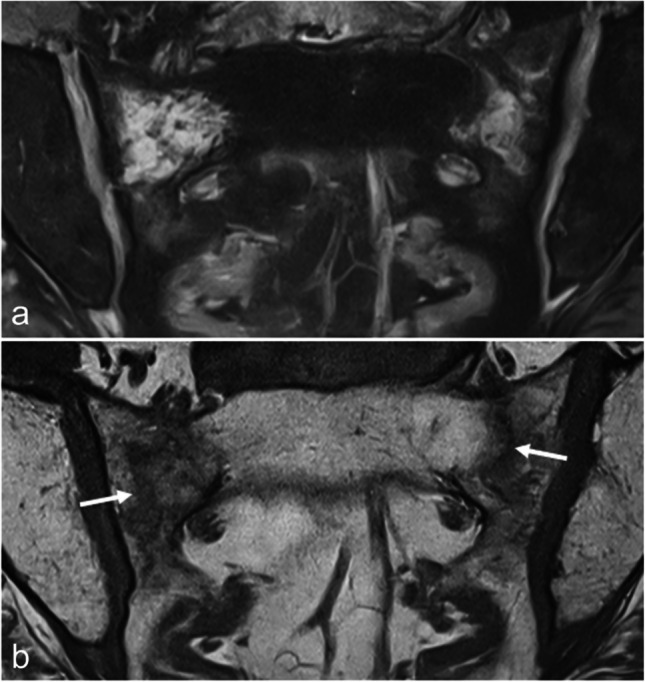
A 72-year-old woman with bilateral sacral insufficiency fractures. Sacral BME is observed on the coronal oblique FS T2-weighted image (a) whereas thin hypointense fracture lines (arrows) paralleling the SIJs are better demonstrated on the coronal oblique T1-weighted image (b)
